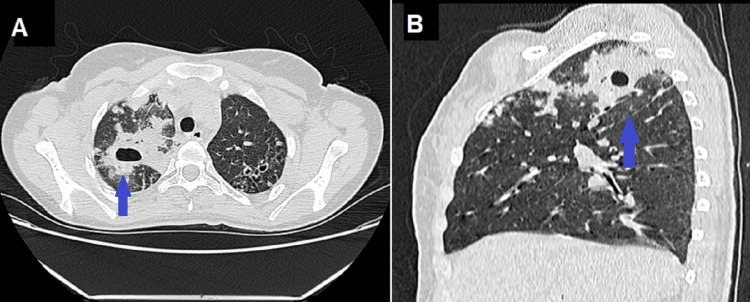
CT scan of the chest: axial (A) and sagittal (B) sections revealing a cavitary lesion with a thick wall in the left lung
